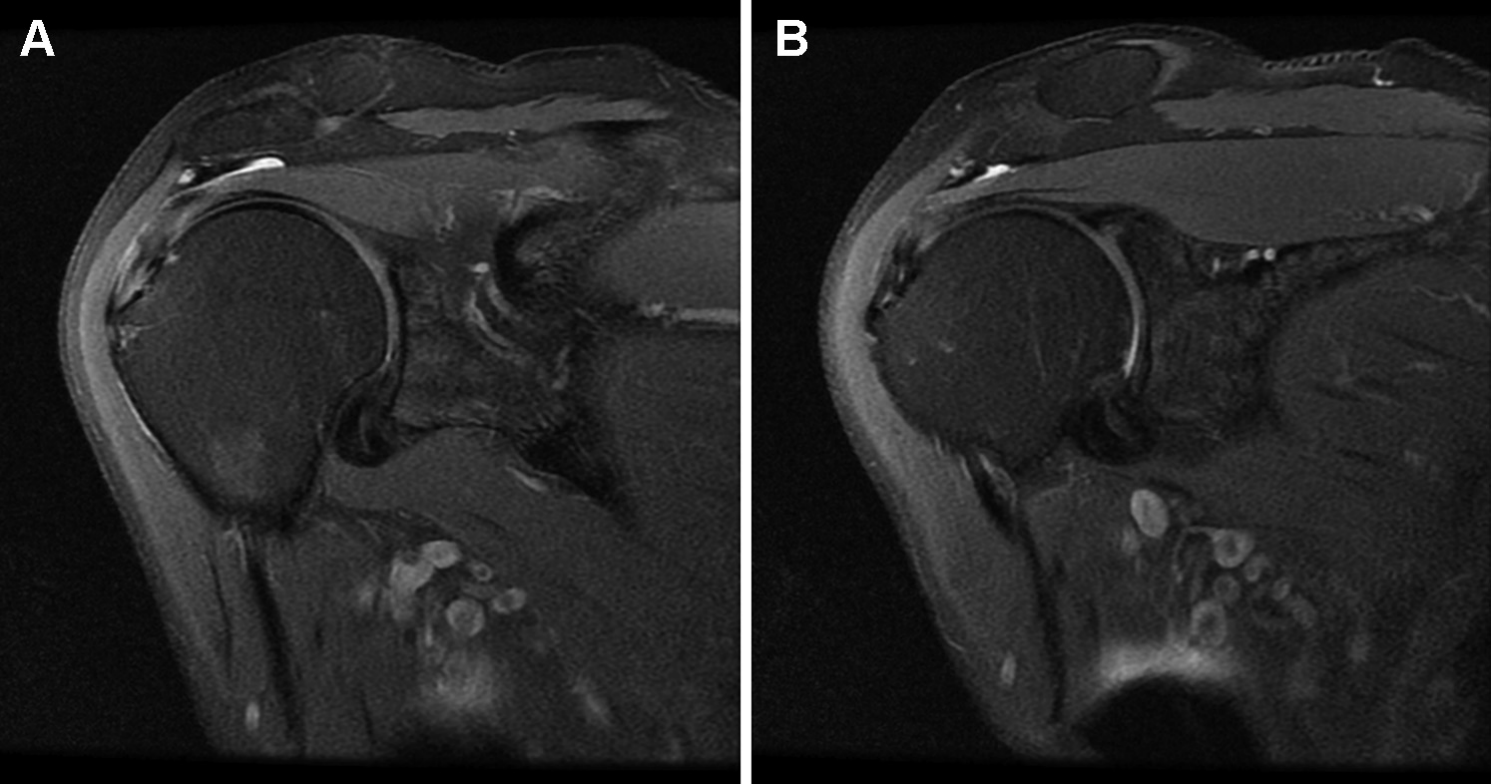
Case one - Proton dense fat-saturated coronal oblique MRI slices of the left shoulder showing high-grade tendinopathy, interstitial tearing, and thickening of supraspinatus and infraspinatus tendons. No evidence of full-thickness rotator cuff tears.
